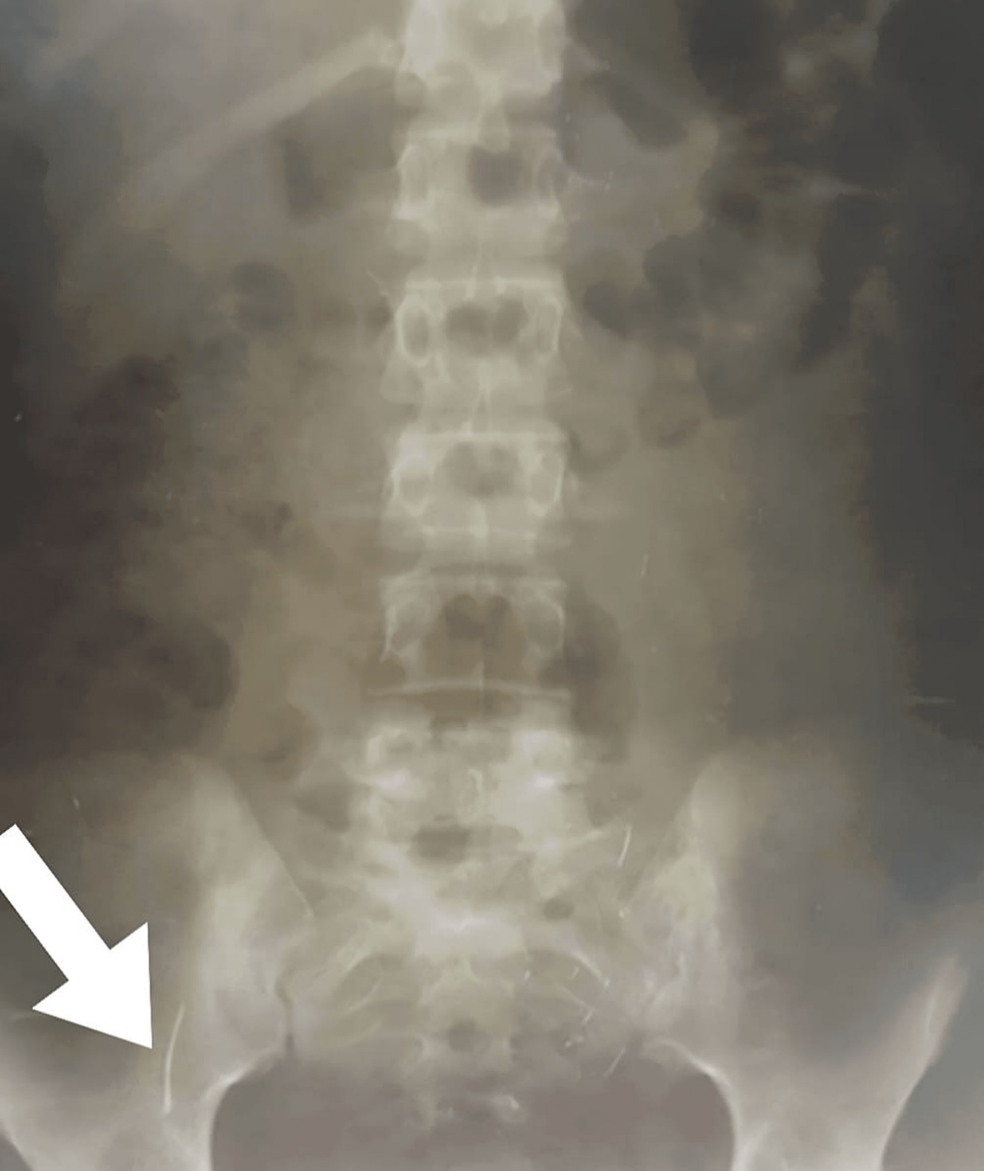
Erect abdomen x-ray. The arrow shows the needle opacity in the appendix.
